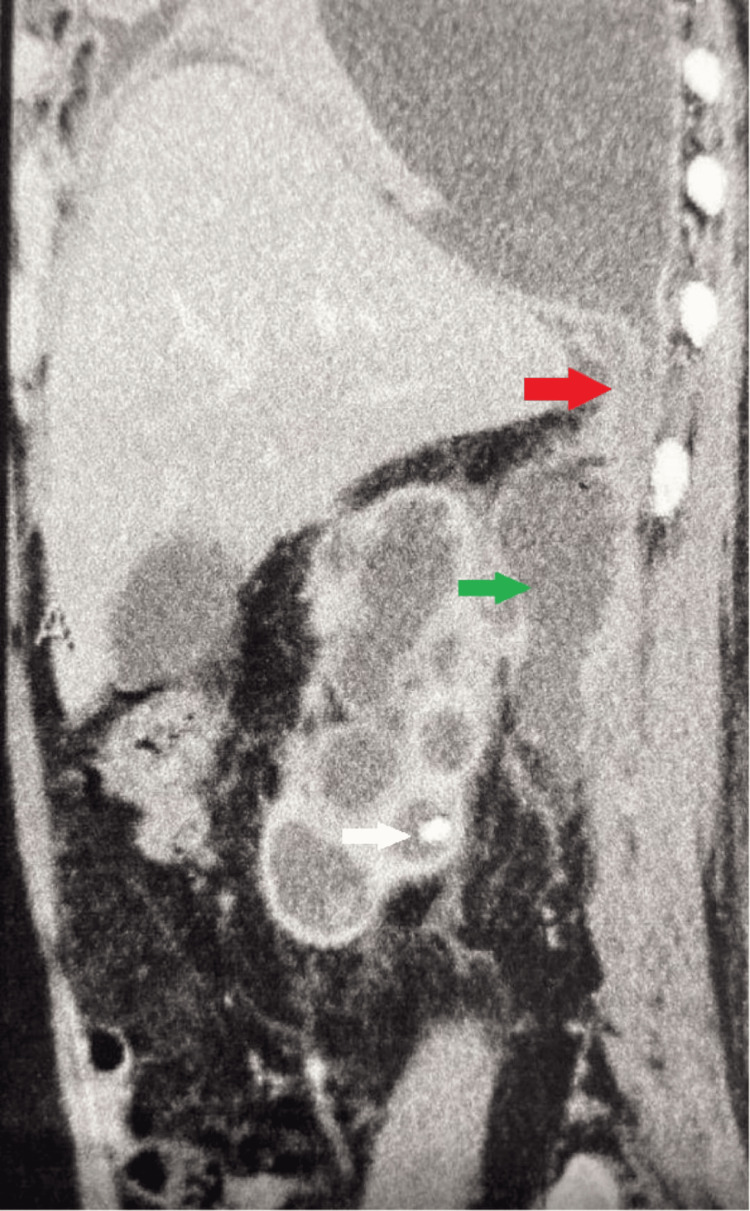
CECT abdomen (sagittal view) - renal calculus (white arrow), perinephric abscess (green arrow), and nephropleural fistula (red arrow) CECT: Contrast-enhanced computed tomography
